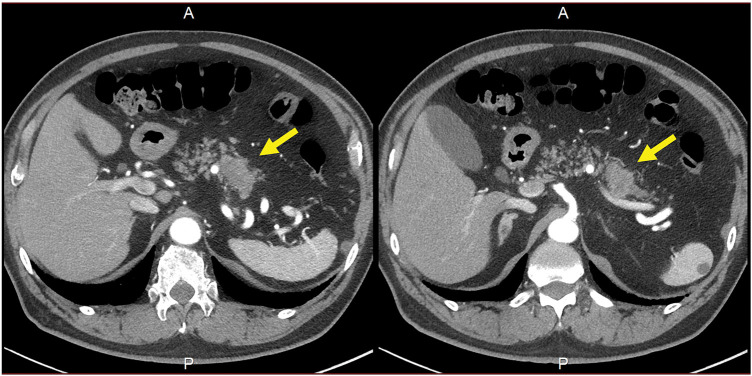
Axial view on contrast-enhanced CT. A 38.6-mm mass in the pancreatic tail can be seen invading the splenic artery (arrows: primary tumor).
